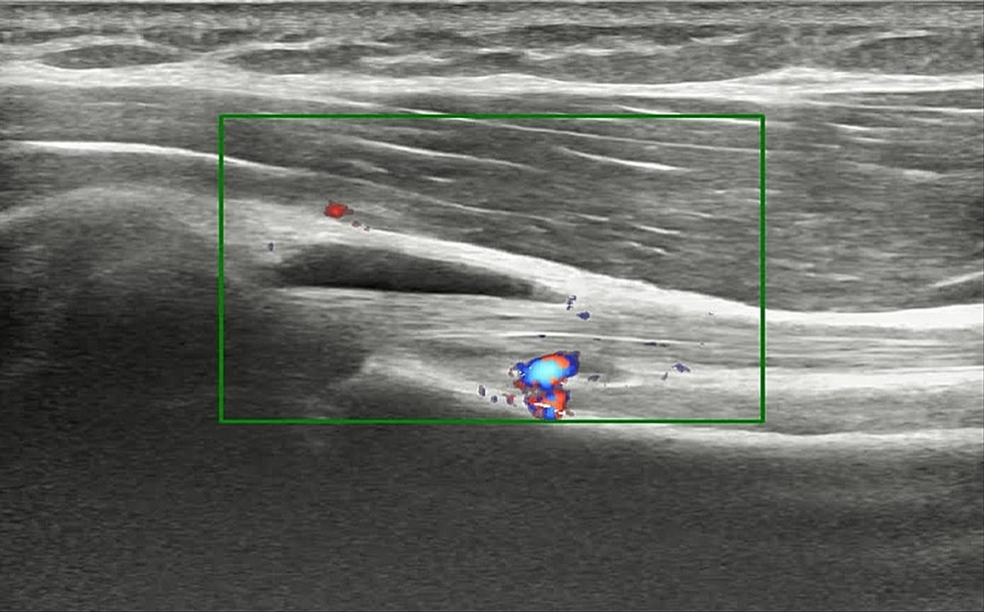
Doppler sonogram showing a negative signal within the lesion.
